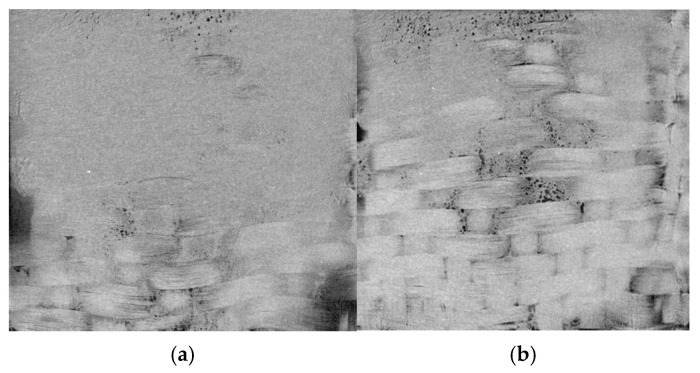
The CT examination of the interlaminar border in US-consolidated laminate; fiber weight fraction 55 wt.% (a) and 70 wt.% (b).
